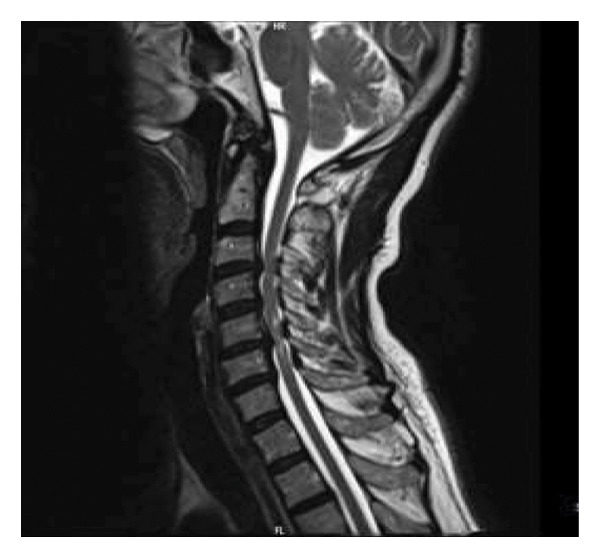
Preoperative T2‐W MRI: disc bulging is seen at C3/C4 and C5/C6 level and signal changes at C5/C6 level are seen in favor of myelopathy.
